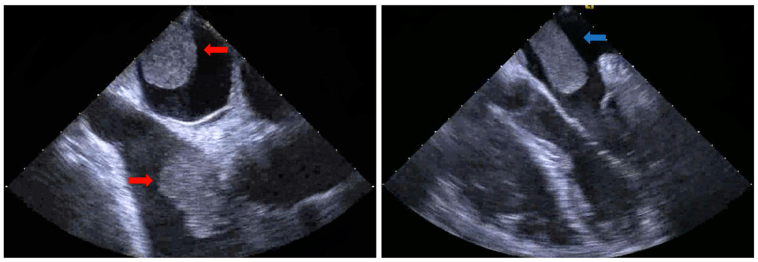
Transesophageal echocardiography. Left: bi-atrial view with both atrial masses (red arrows). Right: left atrial mass (blue arrow).
